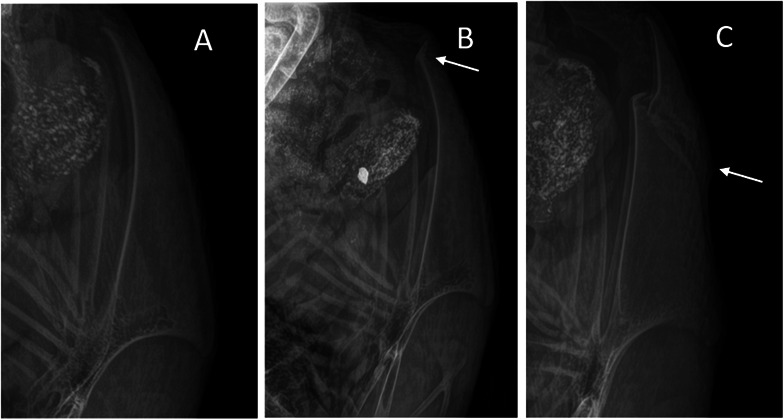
Examples of lateral radiographs taken of a keel bone with (*a*) a score of ‘0’ or ‘no fracture’, (*b*) a fracture score of ‘1.0’ or ‘tip’ fracture where fractures occur close to the caudal end of the keel bone, (*c*) a fracture score of ‘2’ or ‘non-tip’ fracture which includes single or multiple fractures in the non-tip part of the keel and may or may not include tip fractures.
